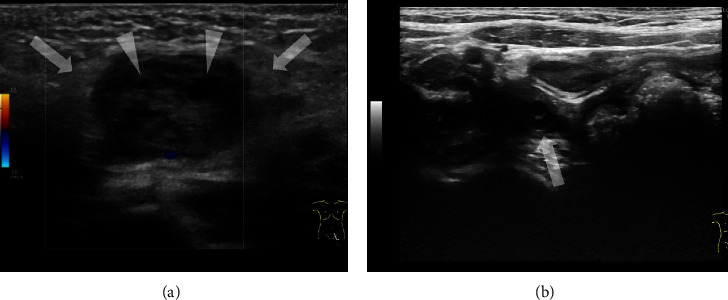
Case 2: a 7-month-old female with herniated ovarian torsion. (a) Sonogram of the left inguinal bulge showing a low-echoic mass with some cystic follicles (arrowheads). Color Doppler imaging shows diminished vascular flow within the mass. The surrounding subcutaneous tissue exhibits edematous changes (arrow). Therefore, herniated ovarian torsion was diagnosed and confirmed surgically. (b) Sonogram of the right ovary. Normal right ovary is detected in the pelvis. A few small cysts from ovarian follicles are evident (arrow).
